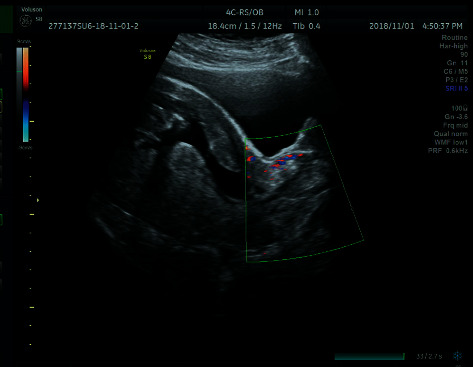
Ultrasonic images of a decidual polyp. A long weak echo was observed in the cervical canal, protruding to the external cervix with a clear boundary. The blood supply seemed to come from the posterior wall of the uterine isthmus.
